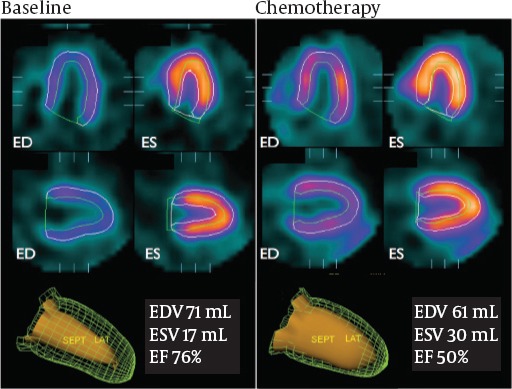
Quantitative gated-SPECT provides cardiac systolic and diastolic functional information. The cardiac function deteriorated in a patient after chemotherapy.
